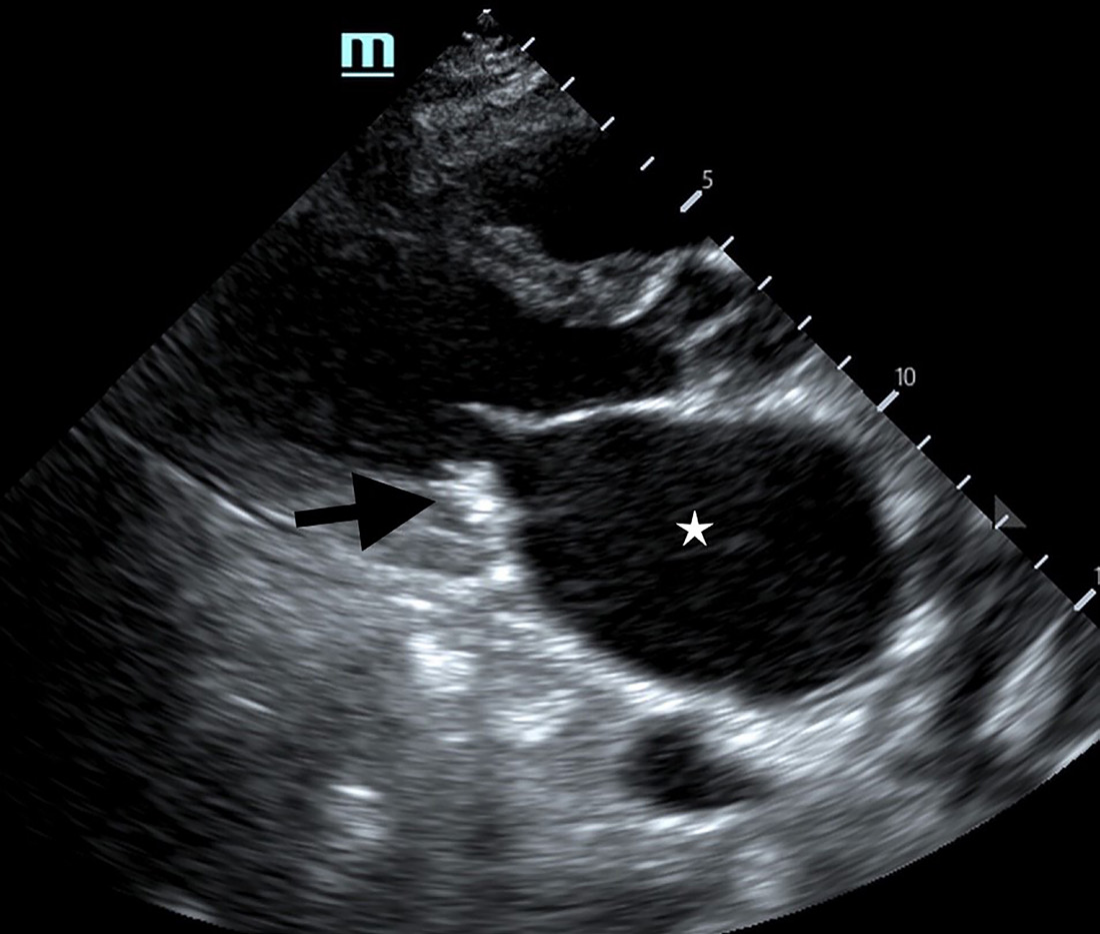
Point-of-care ultrasound in parasternal long view of the heart revealing a hyperechoic structure on the posterior leaflet of the mitral valve representing calcification (black arrow) and left atrial enlargement (white star).
